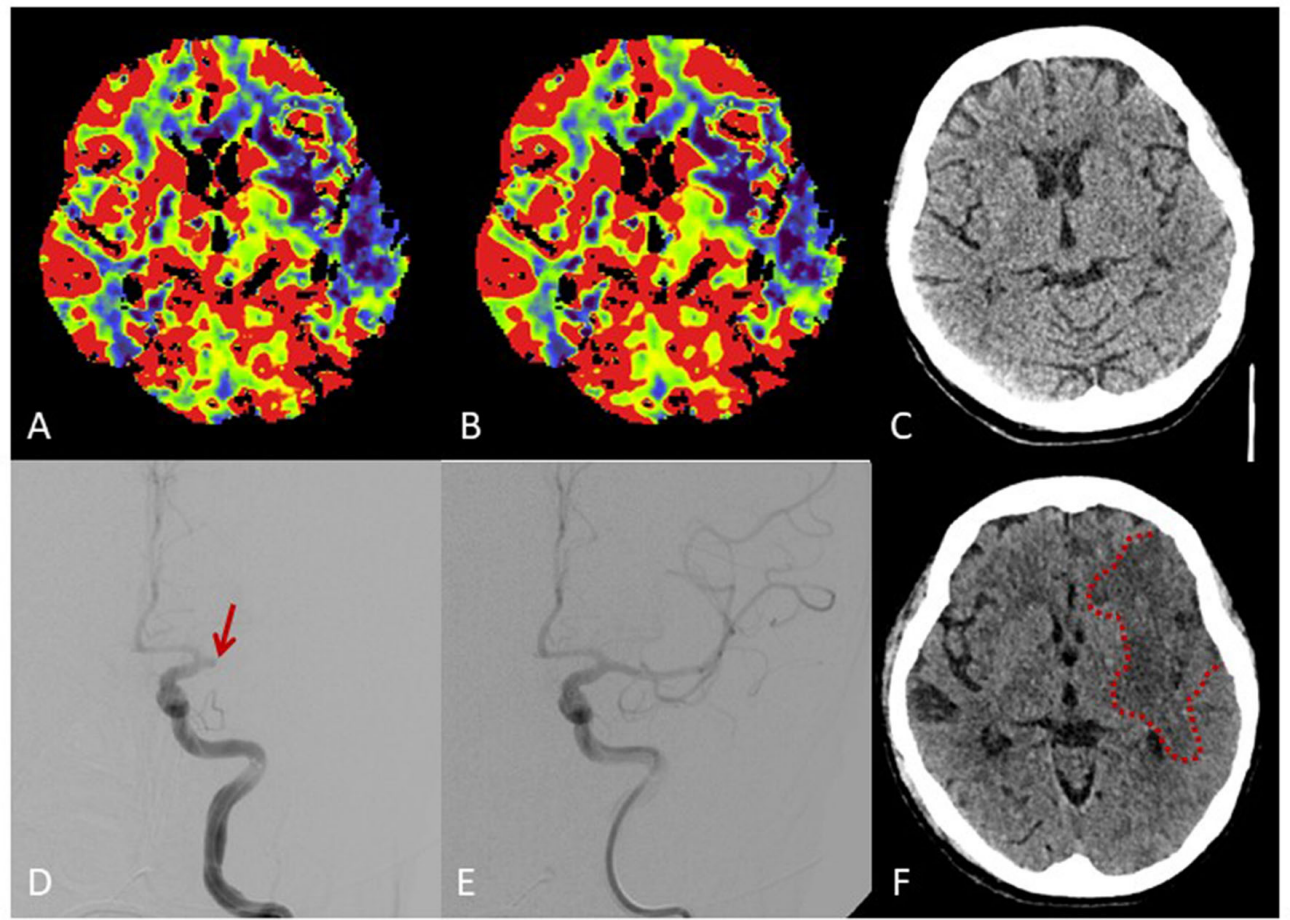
Brain imaging. Multimodal cranial CT imaging at admission shows reduced perfusion in cerebral blood flow maps (A) und cerebral blood volume maps (B) in the territory of the middle cerebral artery (MCA) without early ischemic changes on non-contrast-CT (C). Angiography (D) shows proximal occlusion of the MCA (arrow) with complete recanalization after intravenous thrombolysis and thrombectomy (E). Follow-up CT shows a large frontoparietal MCA infarction despite complete recanalization (F).
